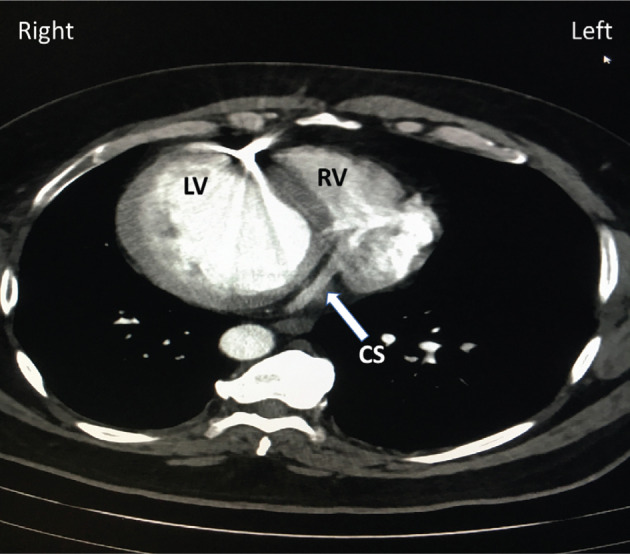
A chest CT scan demonstrating mirror imaging of normal anatomy and dextrocardia. The arrow points to the location of the CS. The right and left sides of the chest are noted. LV: left ventricle; RV: right ventricle; CS: coronary sinus.
